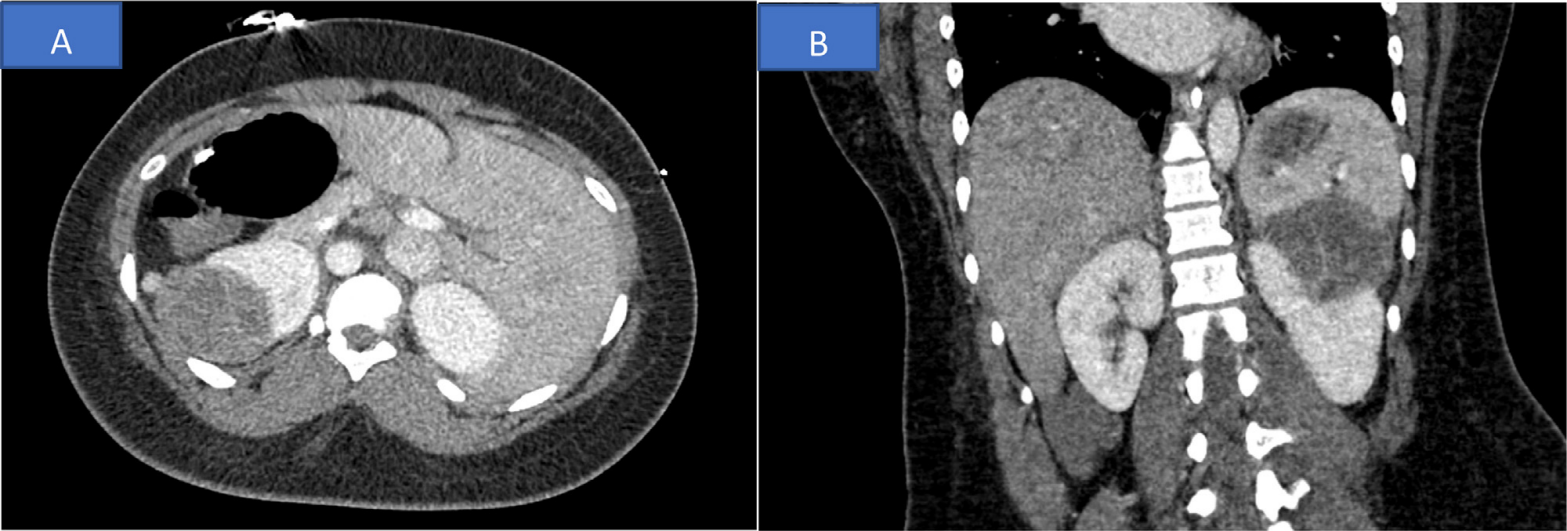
CT scan of the abdomen and pelvis showed a complex left renal mass (6.1 × 5.6 × 5.4 cm) with mixed densities consistent with a probable renal cell carcinoma. (A) Axial view, (B) Coronal view.
